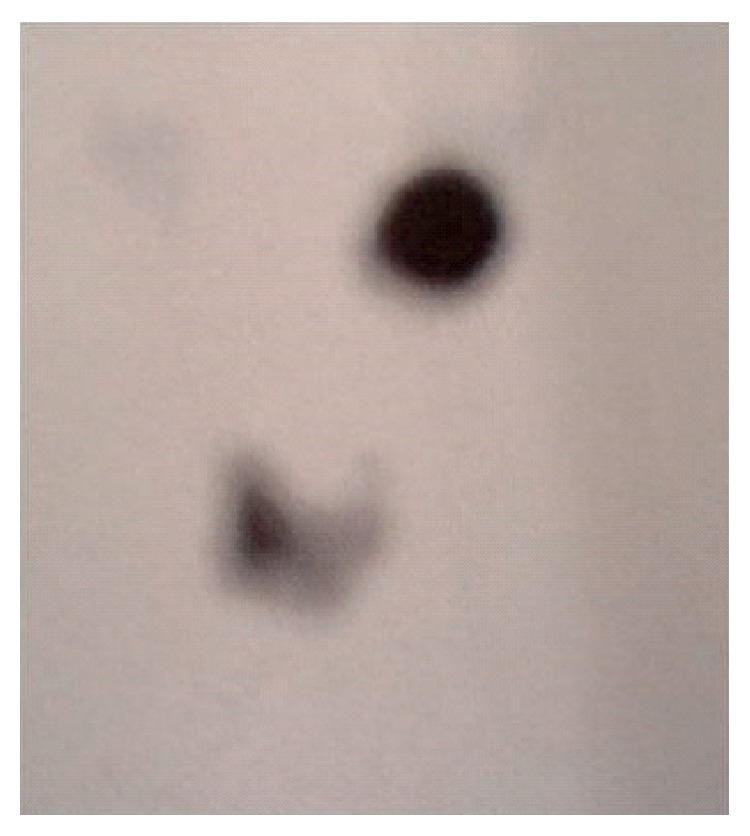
Radioactivity uptake in the normal localization of the thyroid gland and in the submandibular localization on thyroid scintigraphy with Tc-99m pertechnetate.
